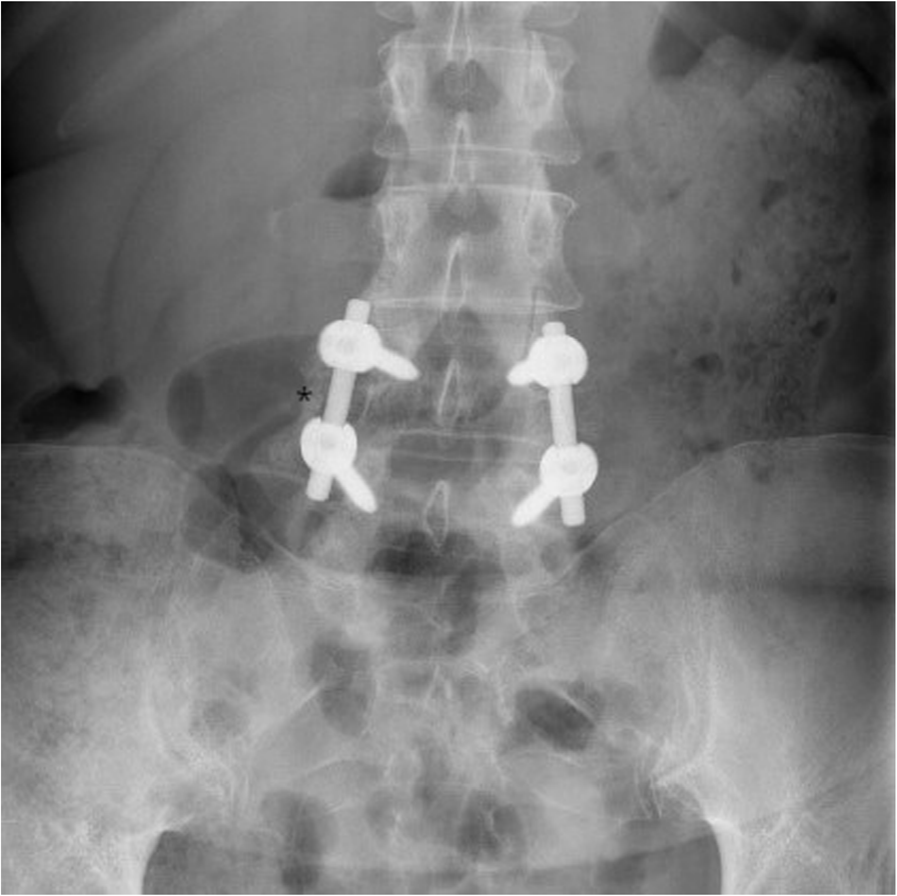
Anteroposterior X-ray of L4–L5 showing bone bridges (asterisk) formation in the intertransverse space for posterolateral spine fusion 1 year after surgery
